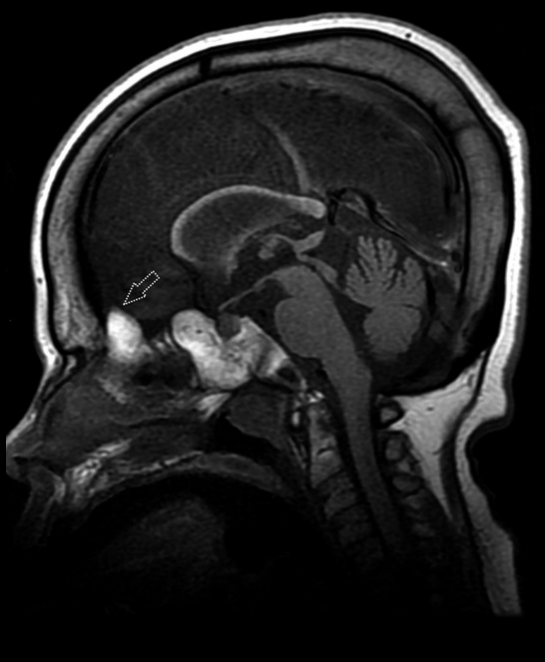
Sagittal T1 weighted MR image shows diffuse calvarial thickening. Note that the lack of pneumatization in frontal and sphenoid sinuses. Hyperintense crista galli has an evident hypertrophic appearance like a white sail (white dotted arrow) (17 years old, MPS type IIIc).
